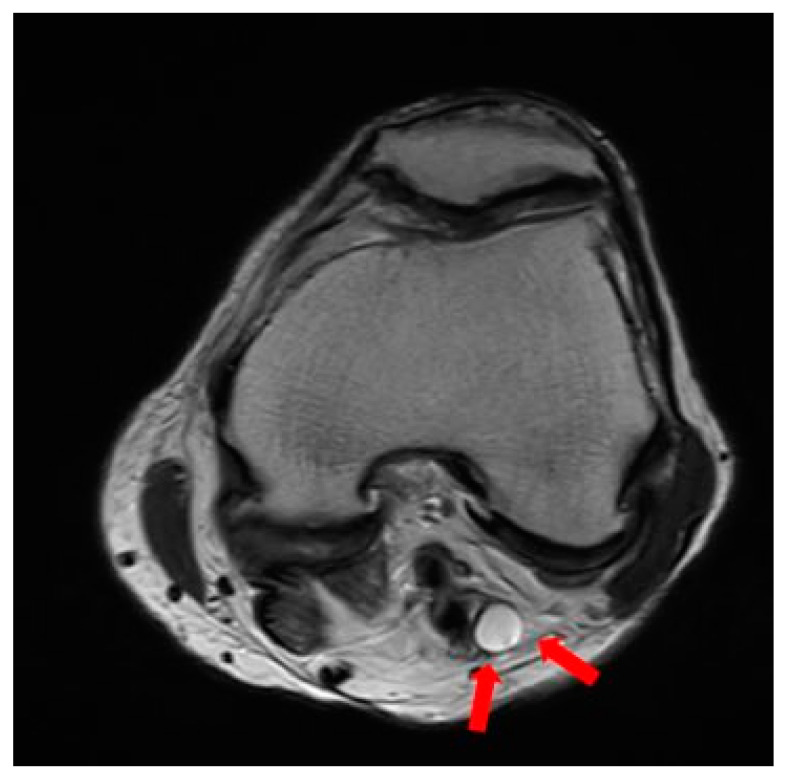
A preoperative knee T2-weighted axial MRI revealing ganglion cysts compressing the base of the tibial nerve (red arrow).
